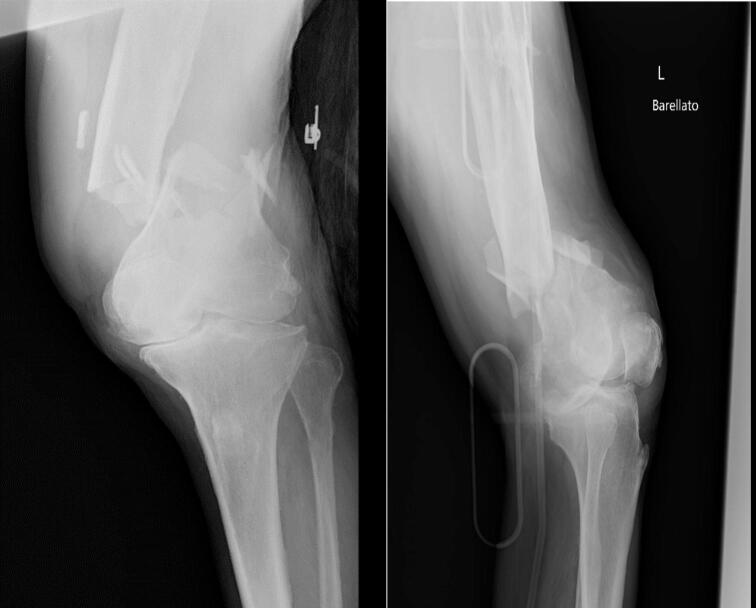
X-rays at the entrance to the emergency room.
